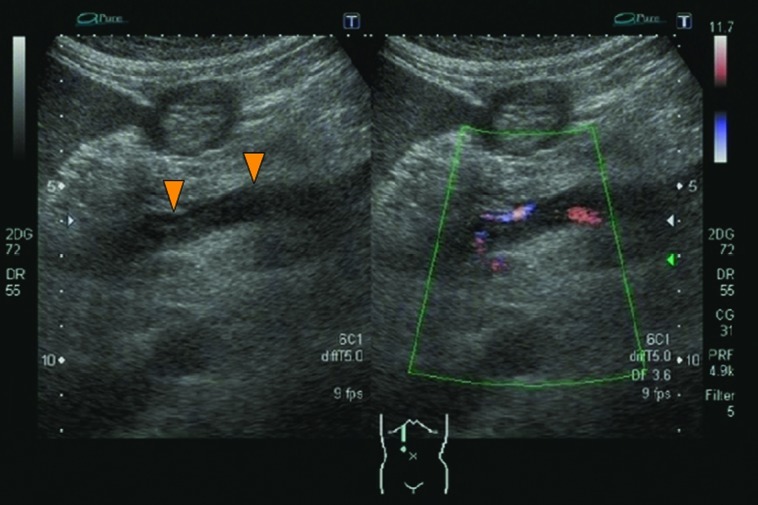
Abdominal ultrasonography (US) findings on admission. Left: B-mode US-detected thrombi (arrowheads) in the superior mesenteric vein. Right: color Doppler US showed neither blood flow through the superior mesenteric vein nor signs of dilatation.
